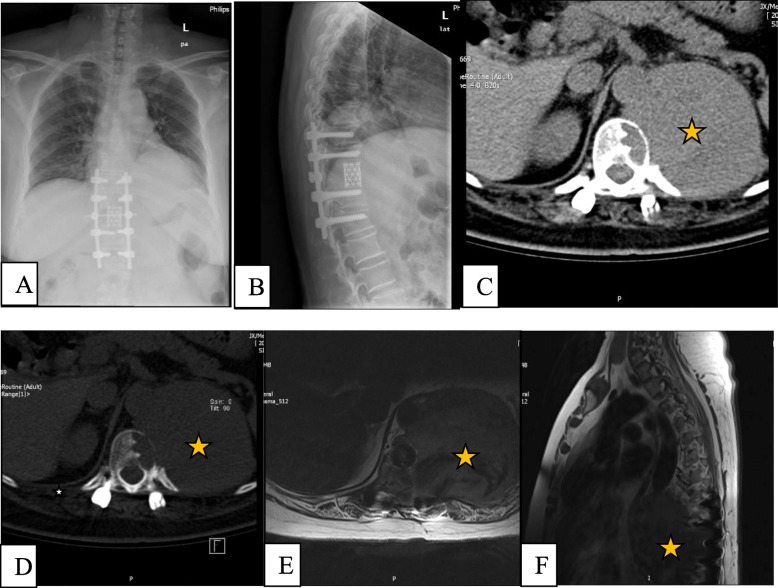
The images of radiograph, CT and MRI for thoracolumbar spine at 20 months after surgery. (a) Anteroposterior and (b) lateral radiographs; (c) and (d) CT axial views; (e) axial T2-weighted MRI; and (f) sagittal T1-weighted MRI. The images show local recurrence of a thoracic spinal giant cell tumour involving the T12 vertebral bodies, as indicated by the orange star
